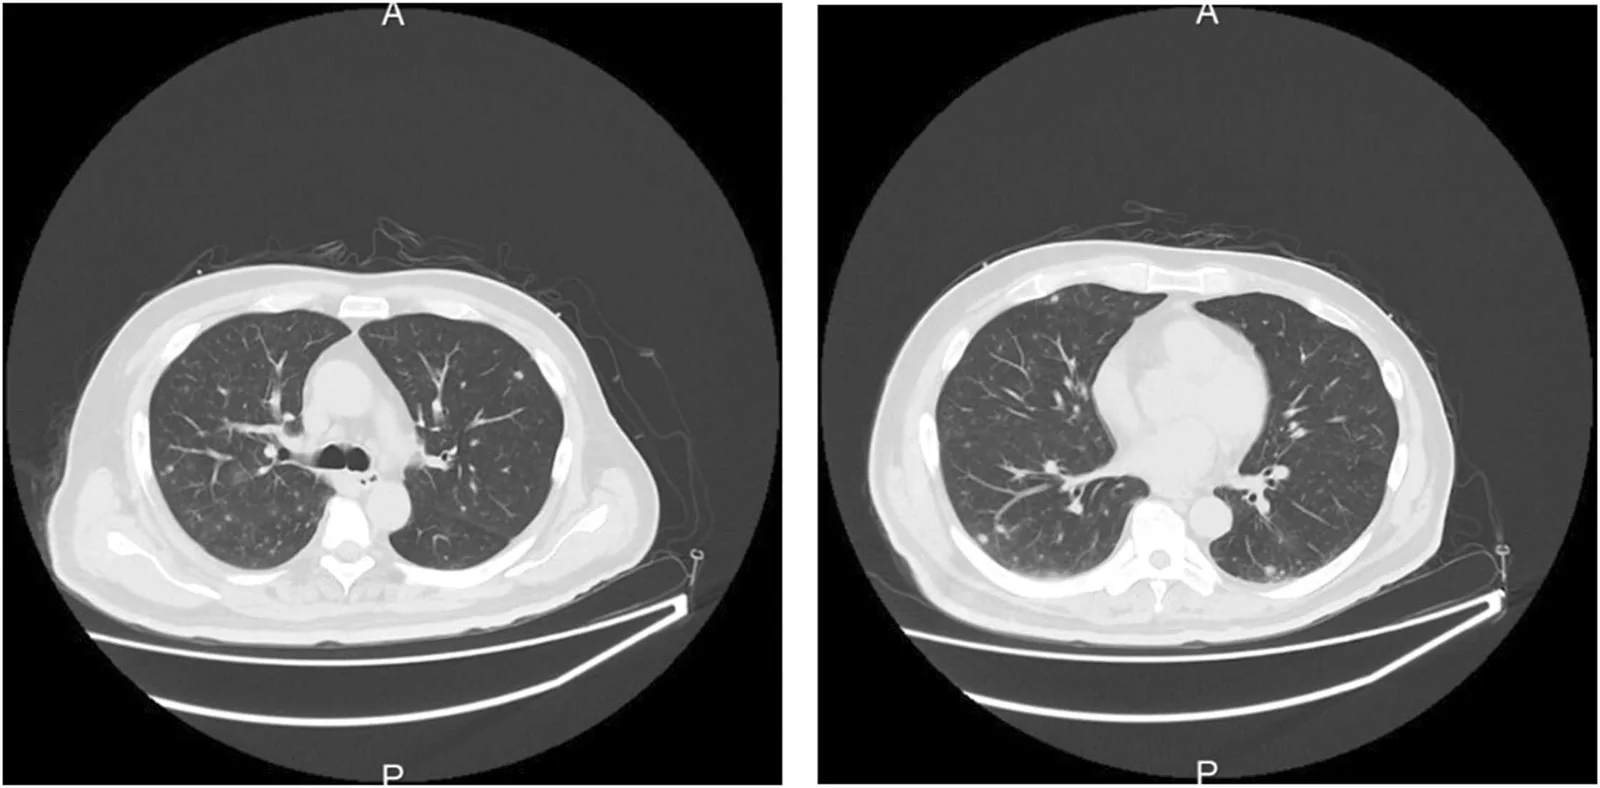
Chest computed tomography at admission revealed bilateral multiple small nodules in a hematogenous distribution.
